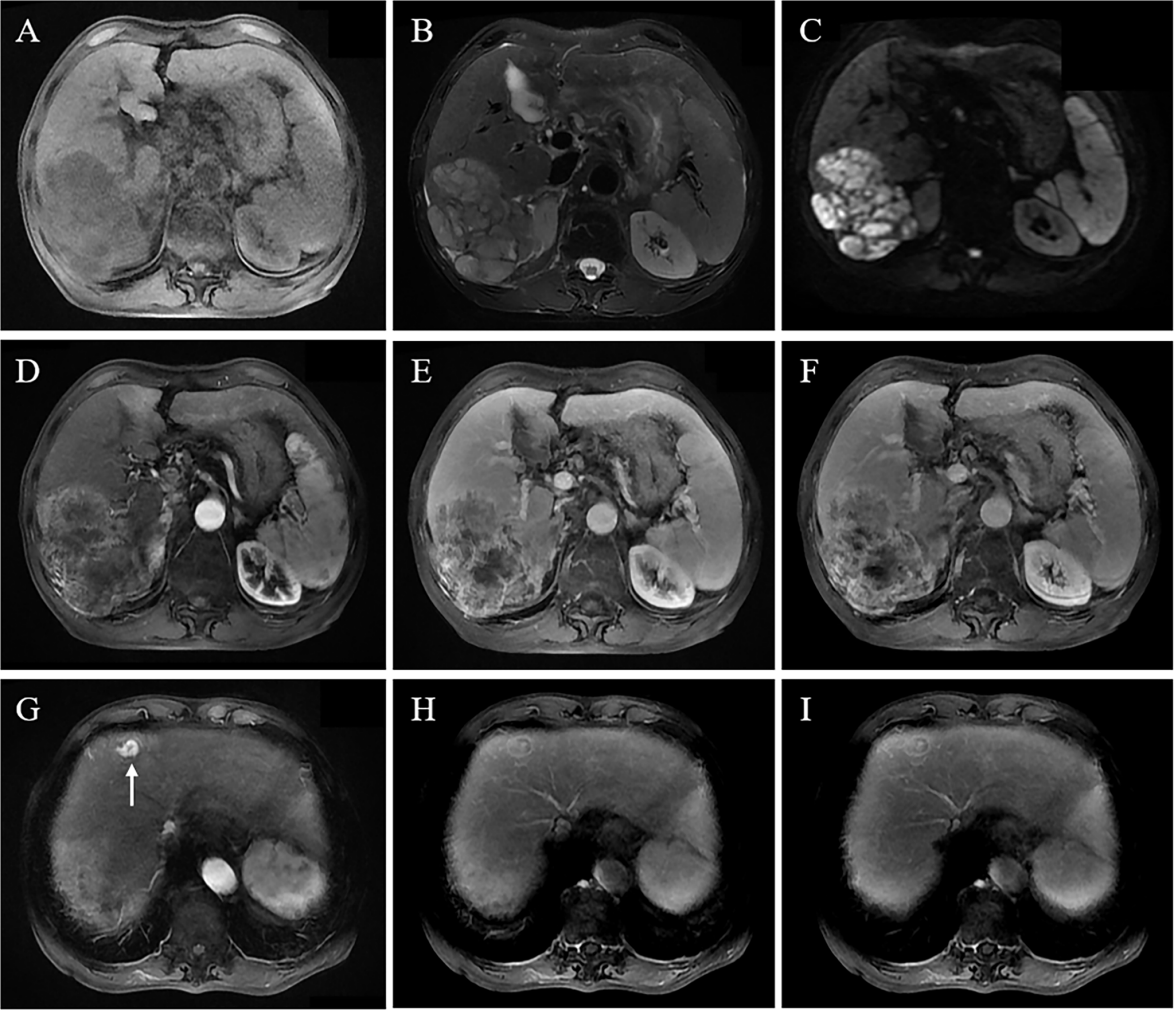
A 68-year-old male patient with chronic HBV and liver cirrhosis underwent an MRI scan because of abdominal pain. It revealed a large mass in the right lobe, 10.5 cm in size. The lesion shows a hypointense signal on T1WI (A), slightly hypointense signal on T2WI (B), and heterogeneously high signal on DWI sequence (C); contrast-enhanced MRI shows heterogeneous enhancement in the arterial phase (D); the lesion shows persistently and heterogeneously high enhancement in portal vein phase and delayed phase (E, F), with persistently high enhancement pattern. Intense enhancement of a small metastatic tumor in the left liver in the arterial phase (H) and persistently high enhancement of a partial lesion in the portal vein phase and delayed phase with an enhanced pseudocapsule (G, I).
